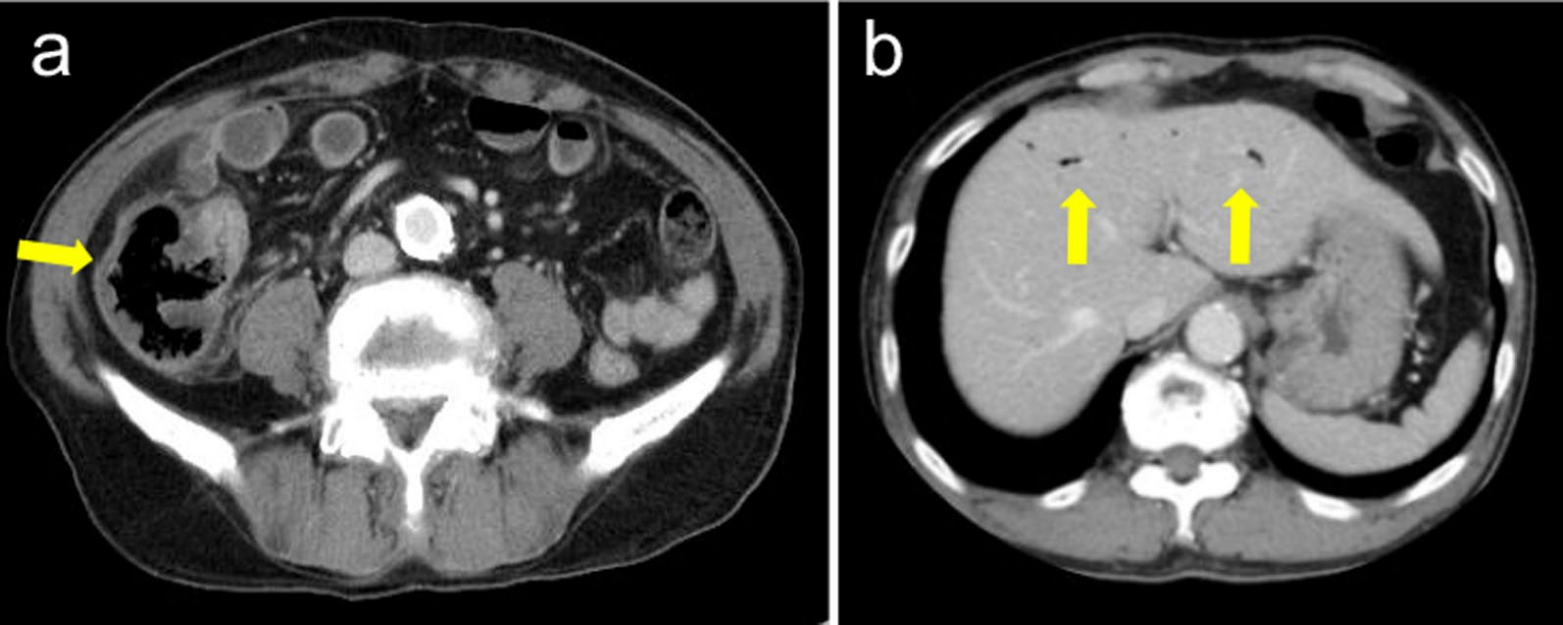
Contrast-enhanced computed tomography. a Enhancement of the cecal wall is attenuated (arrow) and an increase in the surrounding fat tissue density is detected. b Intra-hepatic portal venous gas is observed (arrow)
